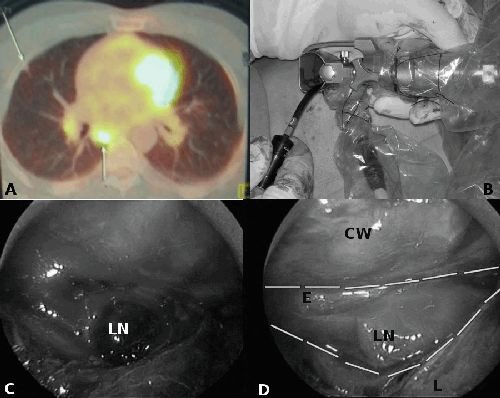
Computed tomography supported positron emission tomography image that identified the fluorodeoxyglucose uptake in the para-esophageal lymph node (A). Image of the video-mediastinoscope used during the operation (B). Lymph node no. 8 can easily be seen by video-mediastinoscope after dissecting the pleural adhesions (C and D). LN, lymph node; E, esophagus; CW, chest wall; L, lung.
